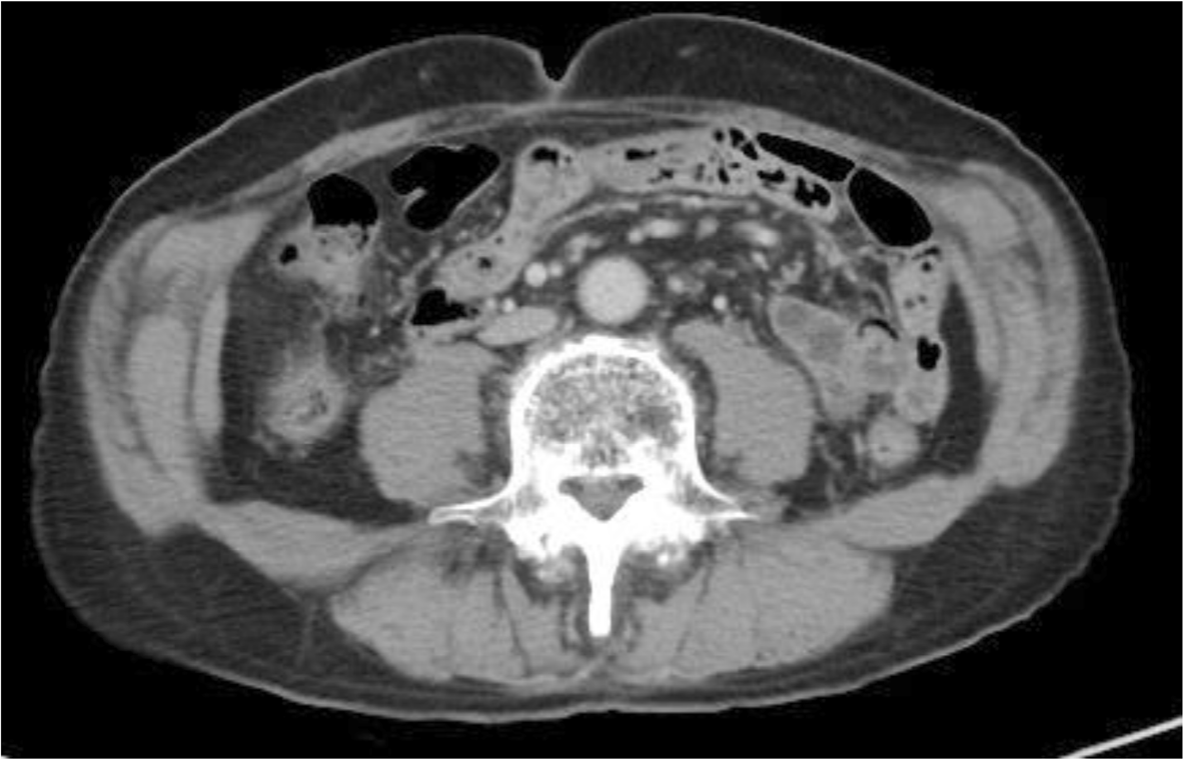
CT performed 28 months after surgery shows increasing subcutaneous fat, indicating improvement in the patient’s health
